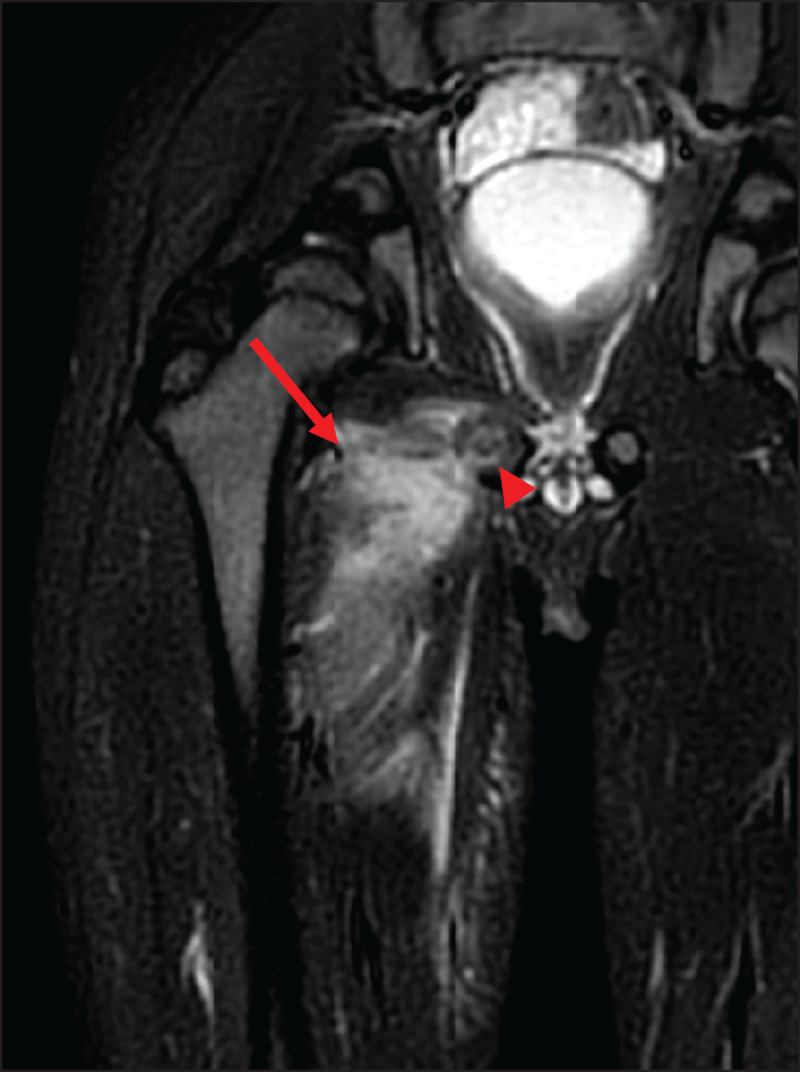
Blood culture revealed a staphylococcus aureus bacteriemia. An antibiotic treatment was administered, and the abscess was monitored with ultrasonography until disapearance, which was confirmed on follow-up MRI six weeks later.
